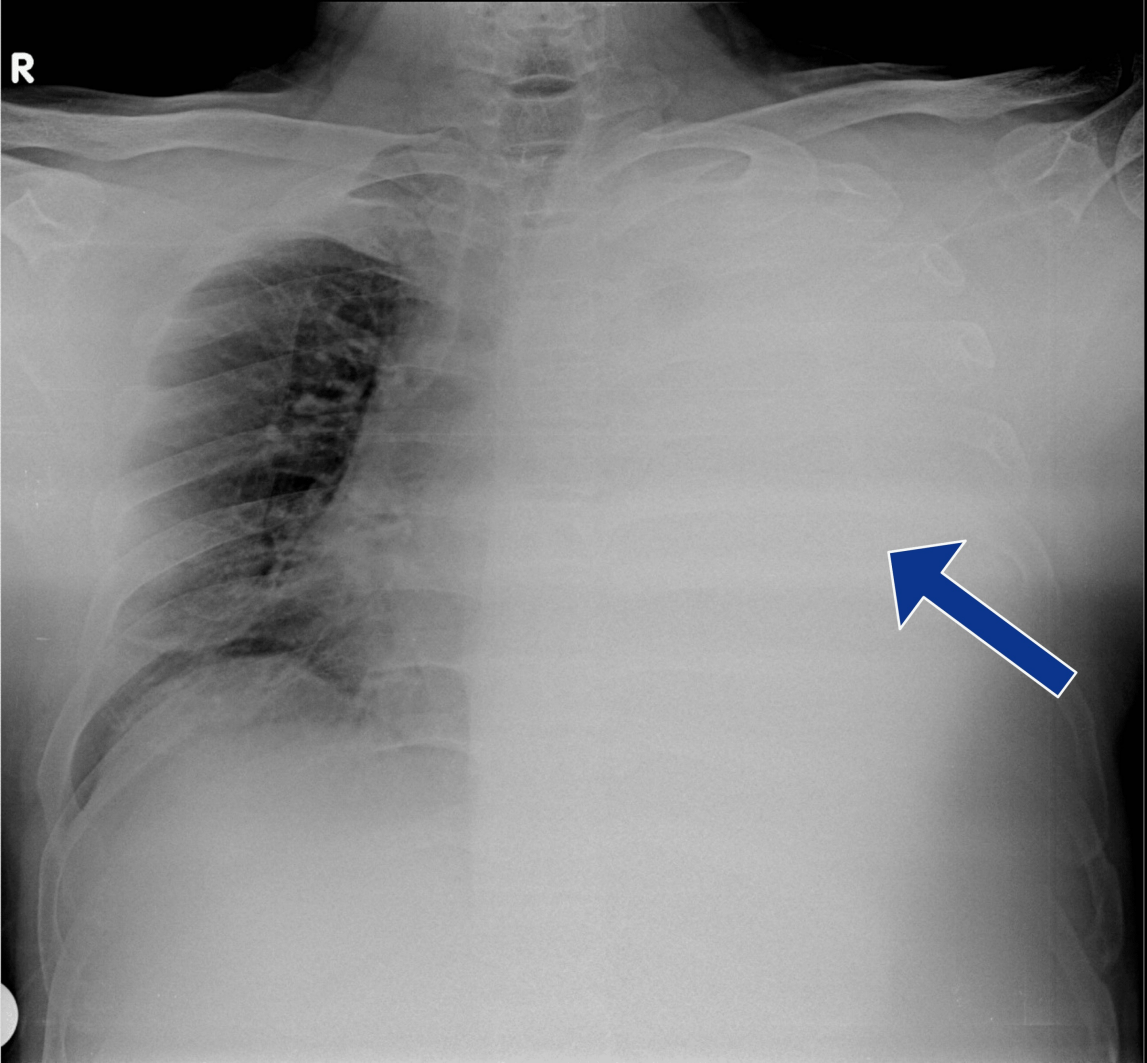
Chest X-ray PA view showing a massive left-sided pleural effusion (blue arrow). PA: Postero-anterior.
